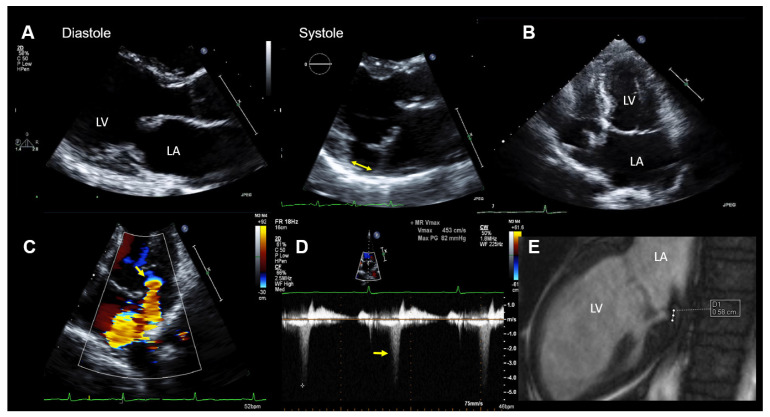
Case 1: Summary of the key findings from multimodality imaging. (A) The mitral annular disjunction (MAD) (yellow double arrow) is only visible in systole in a parasternal long-axis transthoracic echocardiography (TTE) view. (B) A mildly enlarged left atrium is seen in a 4-chamber TTE view. The MAD is not clearly visualized. (C) The proximal isovelocity surface area (PISA) zone (yellow arrow) is seen. A PISA radius of 4 mm and a vena contracta of 3.5 mm indicated nonsevere mitral regurgitation (MR). (D) MR occurs only in the late-systole (yellow arrow) in Doppler imaging. (E) The MAD is measured in cardiac magnetic resonance imaging (white dots = 5.8 mm). LA = left atrium and LV = left ventricle.
